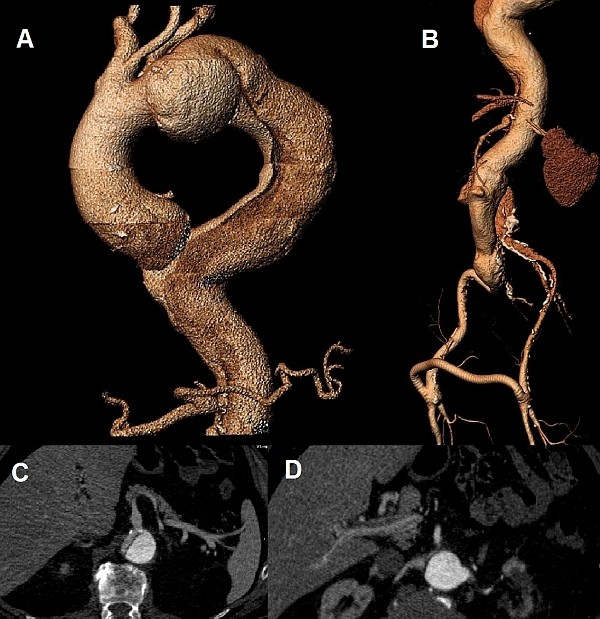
Pre-operative images of the aorta. (a.) 3D CT reconstruction demonstrating the dissecting type B aneurysm and the dilatation of the arch and ascending aorta (b.) 3D CT reconstruction image of the dissected abdominal aorta and the patent femoro-femoral bypass graft (c.) Axial CT image showing the origin of the celiac trunk from the true lumen (d.) Axial CT image demonstrating the origin of the left renal artery from the false lumen and right renal artery from the true lumen.
